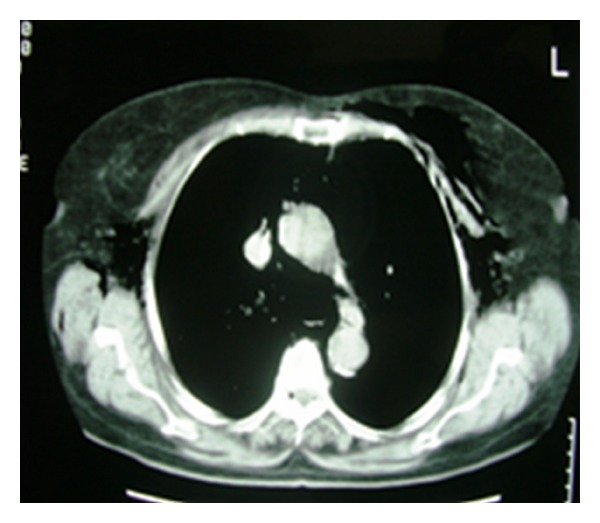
Transverse CT image of the chest revealing bilateral diffuse subcutaneous emphysema and pneumomediastinum. Free air is noted at the anatomic region of the aortic arch and the descending thoracic aorta.
